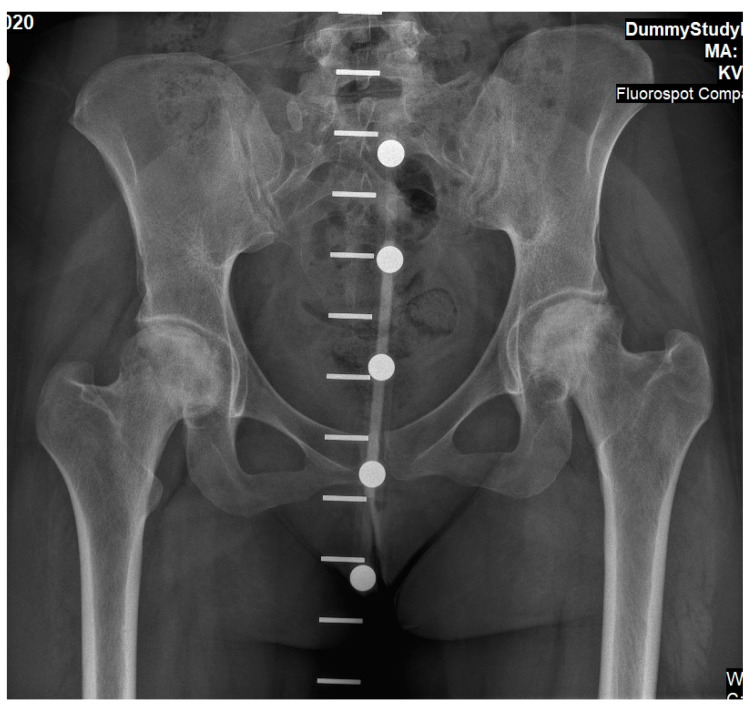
An antero-posterior X-ray of pelvis and hip joints of Case 2. This was taken as part of pre-operative planning for simultaneous bilateral hip arthroplasty (note the calibration marks used for implant selection). At this stage she was in severe pain. These findings present osteonecrosis changes to femoral heads, although sphericity is partially preserved and joint space is still evident.
